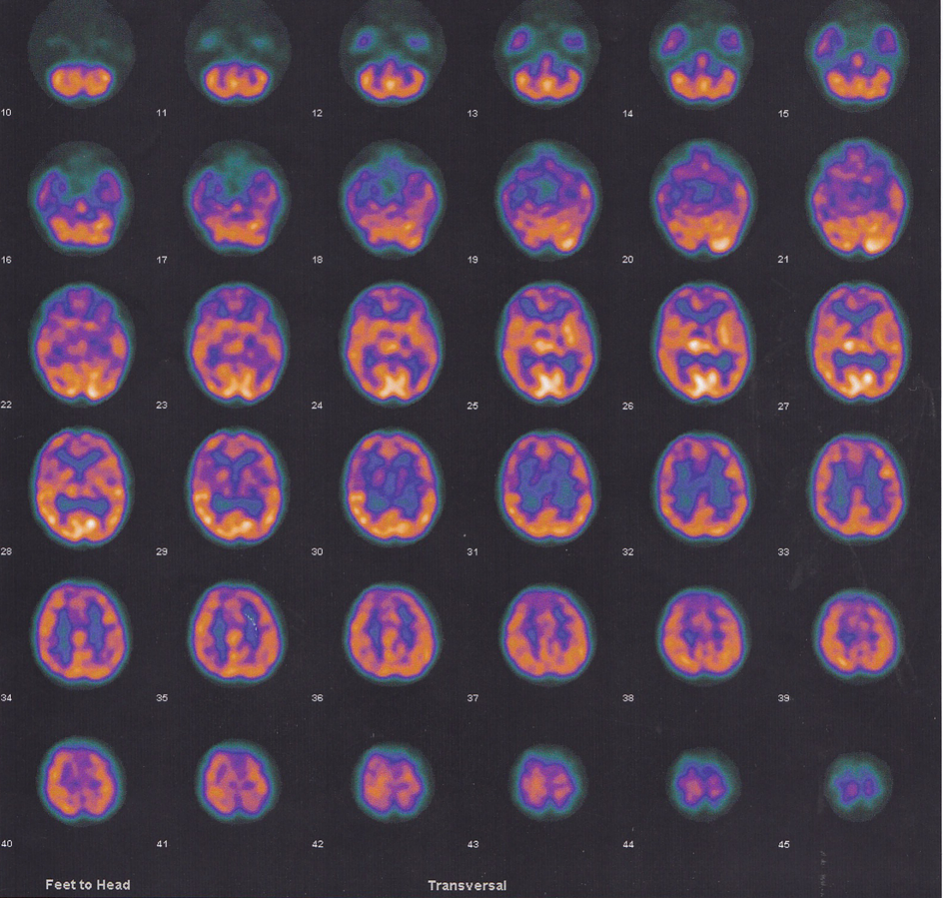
Interictal brain SPECT with 99mTC-ECD showed a cerebral hypometabolism in both right and left parietal and occipital lobes.
